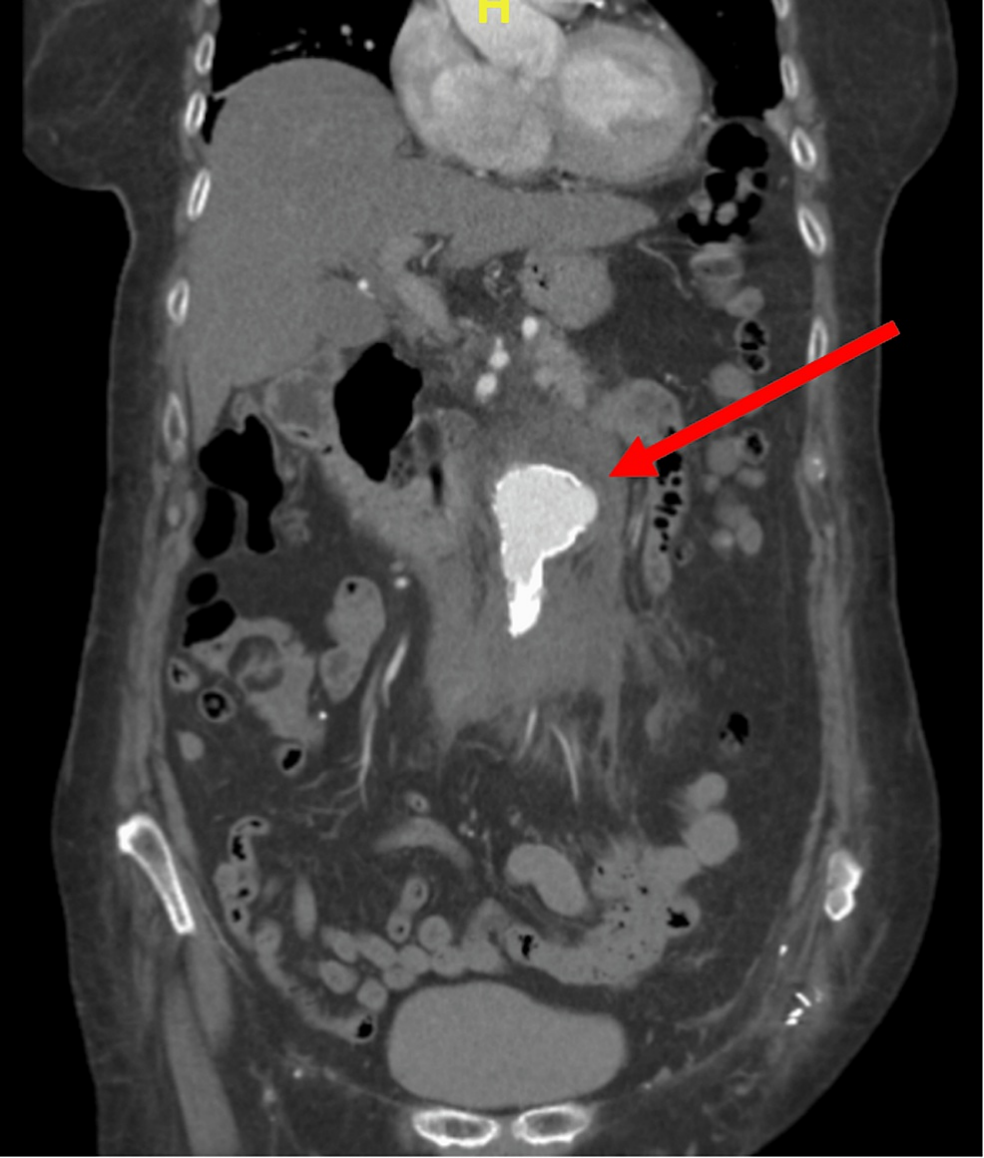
Coronal view CTA demonstrating aortic ulceration with pseudoaneurysm and intramural hematoma extending through the aortic wall
